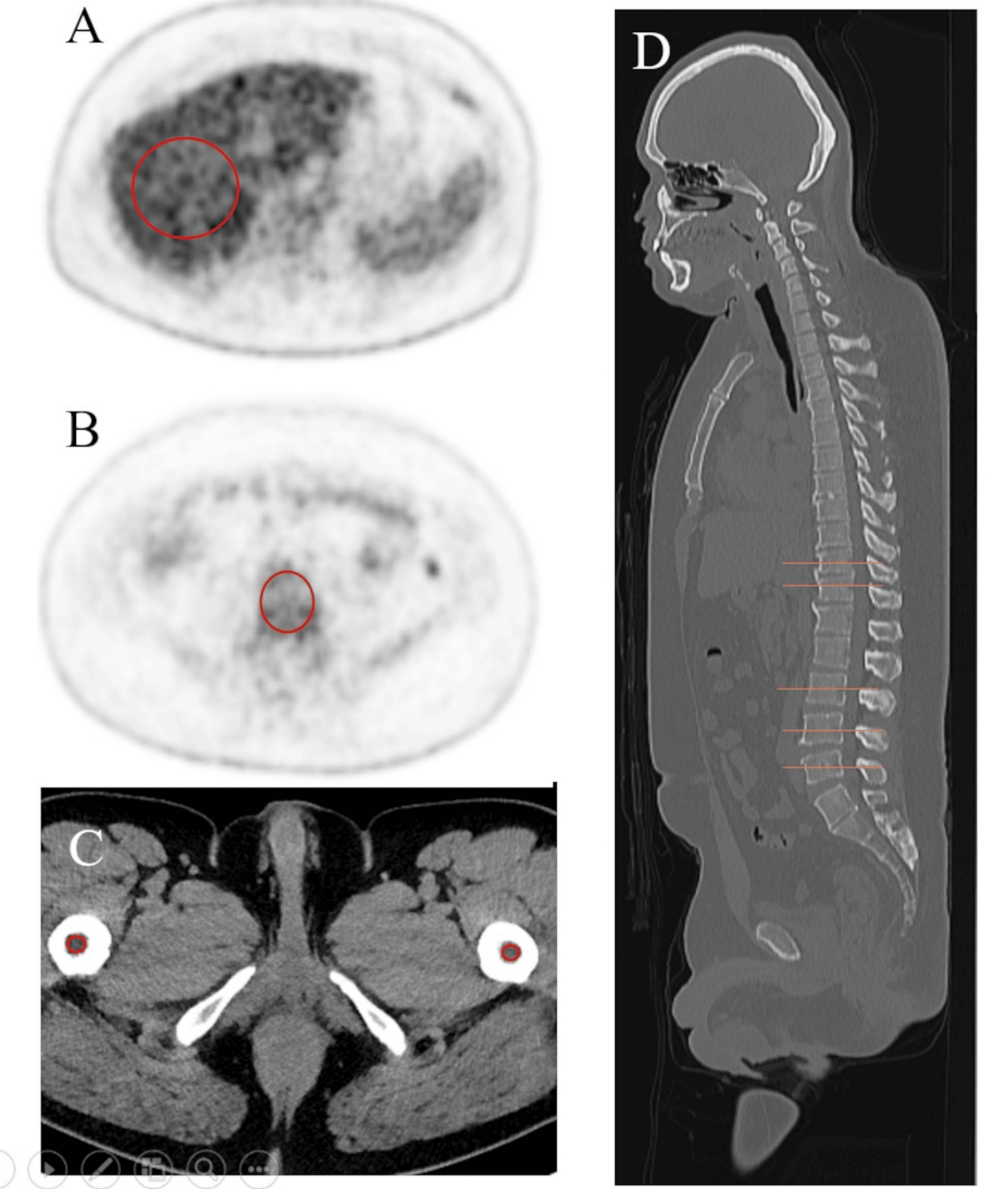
Placement of regions of interest (ROI). The ROIs were placed at the center of the Th11, Th12, L3, L4, and L5 vertebrae (B), using the scout tool (D), and the obtained mean standardized uptake values (SUVs) were averaged (Vertebral-SUV). The elliptical ROI of the liver was placed on the middle part of the right lobe (A), and the mean SUV was measured (Liver-SUV). Bone marrow-to-liver ratio (BLR) was calculated by dividing the Vertebral-SUV by the Liver-SUV. The ROI for the femoral bone marrow (C) was placed on the caudal side of the lesser trochanter, where the trabecular bone was not visible, and both sides of the mean Hounsfield units (HU) were averaged (BM-CT number).
